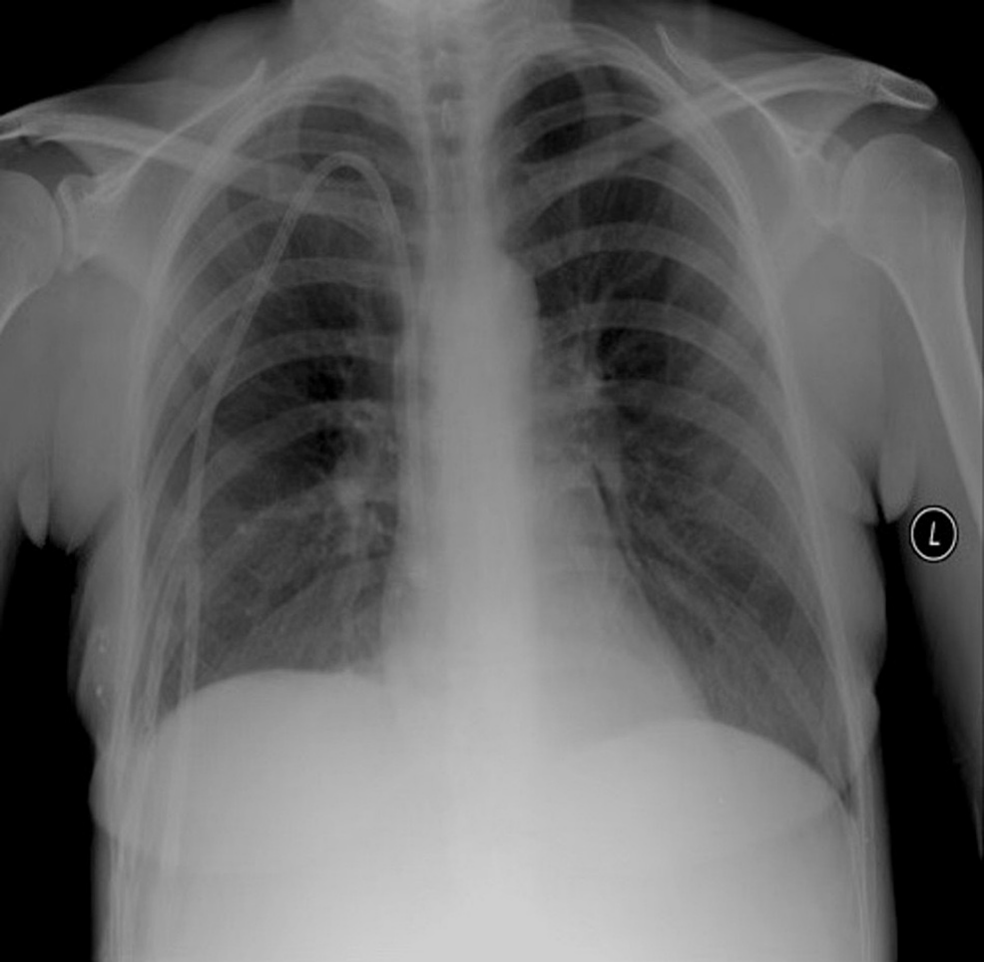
Normal chest X-ray dataset example
